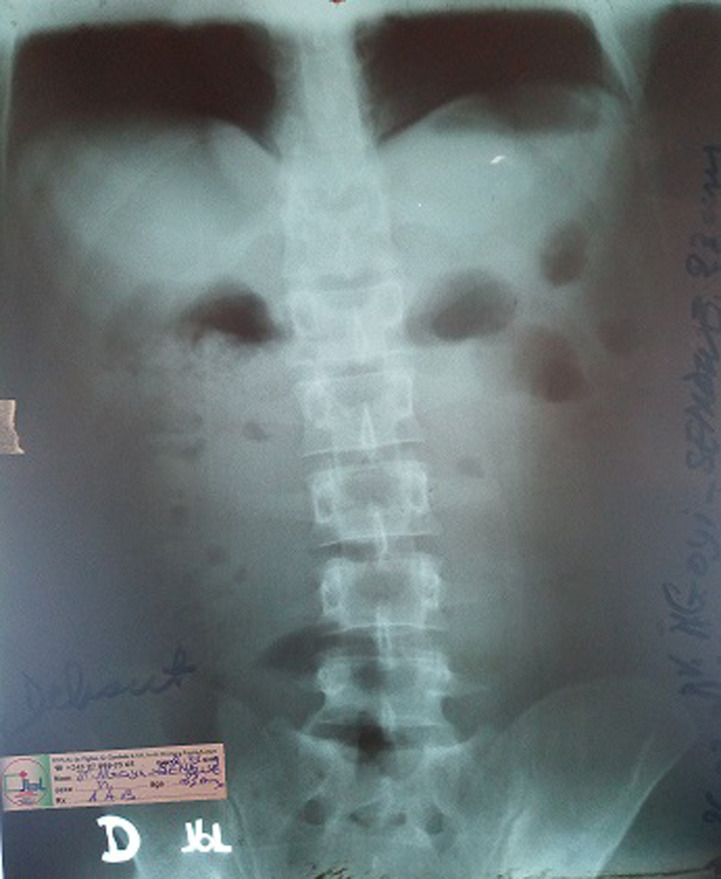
radiographie abdomen à blanc
